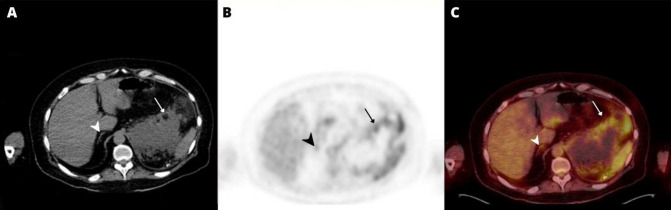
Axial CT (A), corresponding PET (B), and fused PET/CT (C) images revealed an irregular soft tissue mass of 14 cm in the left suprarenal region with mild non-specific FDG uptake (arrows), which was confirmed by biopsy to be negative for malignancy afterward, as well as normal morphology and metabolic activity of the right adrenal gland (arrowheads)
